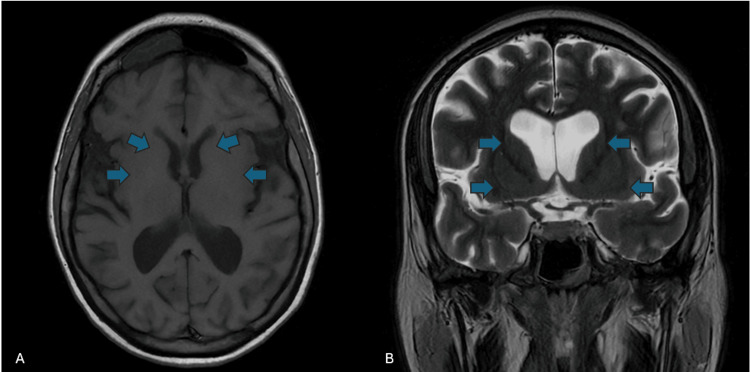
MRI brain on day one of admission (A) displaying axial T1 image with microvascular changes but no hyperintensities or focal abnormalities and (B) displaying coronal T2 images without hyperintensities or focal abnormalities
